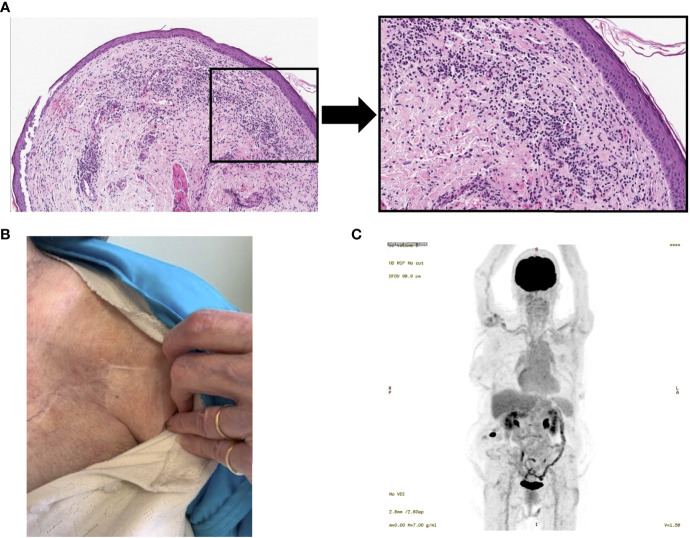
Resolution of cutaneous metastasis following intralesional IL-2 and topical imiquimod. (A) Punch biopsy of injected site at the 14-week timepoint, 100X magnification (left) and representative section, 200X magnification (right) showing no evidence of adenocarcinoma, with chronic immune infiltrates. (B) Image of lesion post-treatment, showing complete clinical response. (C) Post-treatment PET-CT (2 weeks after the final treatment; week 34) revealing no evidence of PET-avid lesions overlying the left pectoral muscle.
